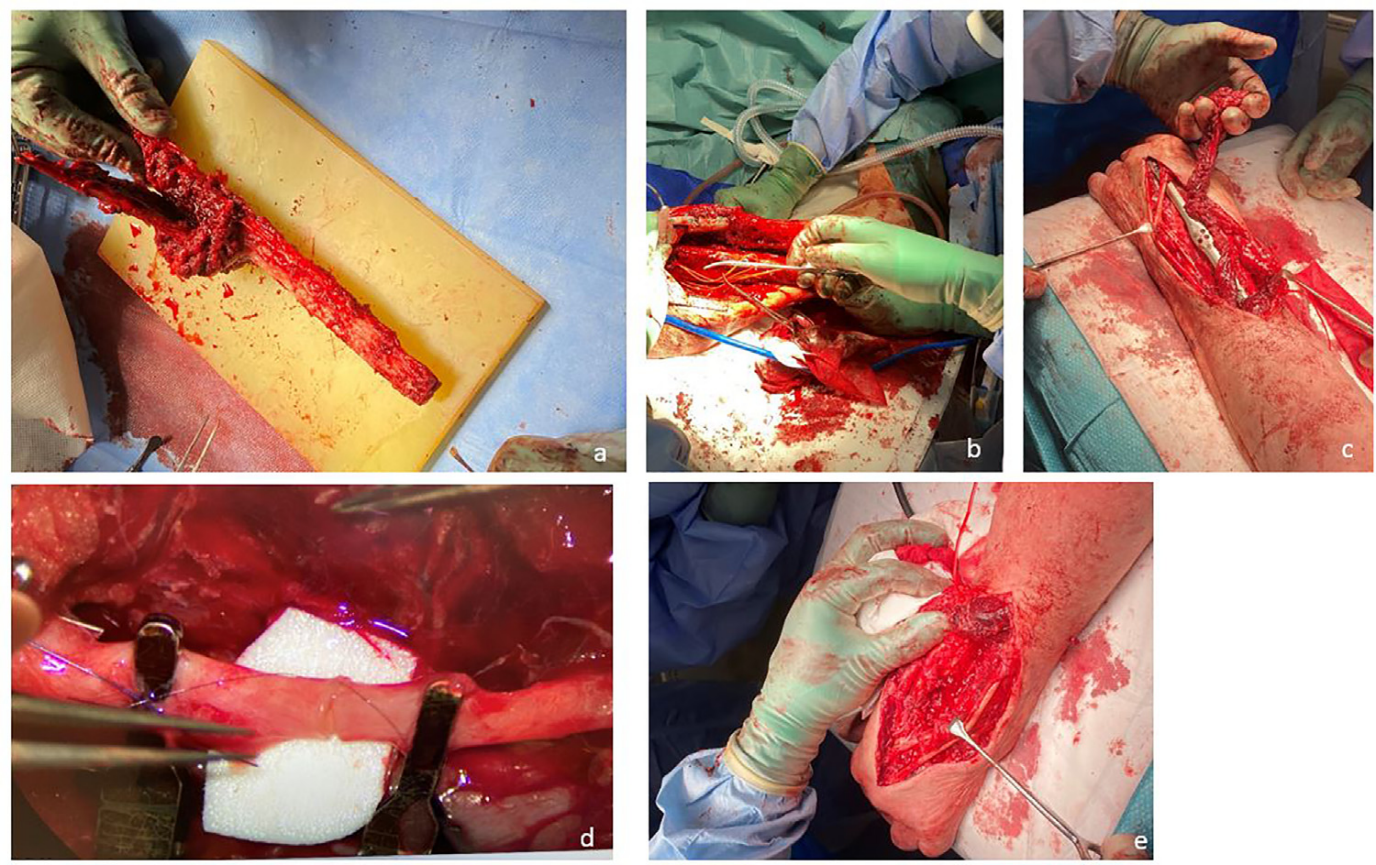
a) CT angiography underarm; b) CT angiography lower leg.
